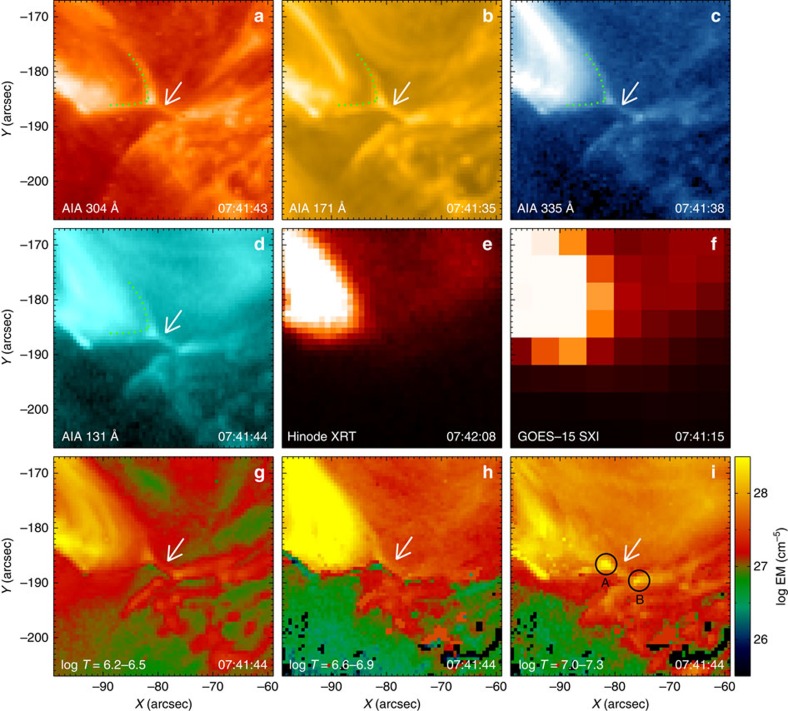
EUV/X-ray images and EM maps. (a–d) AIA EUV images at 304, 171, 335 and 131 Å channels, in which the cusp-shaped structures are marked by the dotted lines and the current sheet is indicated by the white arrows. (e,f) Cusp structure seen in X-ray images observed by Hinode/XRT and GOES/SXI, respectively. (g–i) EM maps in three temperature ranges showing the dominant temperatures of the inflow region, current sheet, cusps (encircled and marked ‘A' and ‘B' in i), and reconnected loops.
